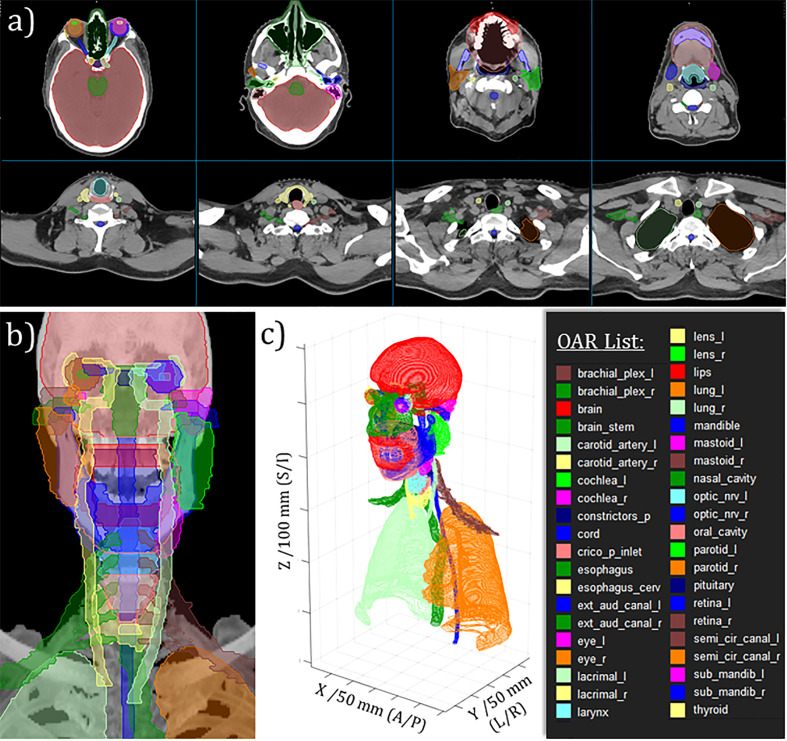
Example of curated H&N OAR segmentations for randomly selected case. (A) Moving from left-to-right, top-to-bottom: selected axial slices moving from superior to inferior aspect of H&N RT planning CT. (B) Anterio-posterior maximum intensity projection rendering with OAR projections overlayed. (C) 3D perspective rendering of 2D DICOM-RTStruct-format OARs with tick-marked scales on DICOM coordinates as indicated.
